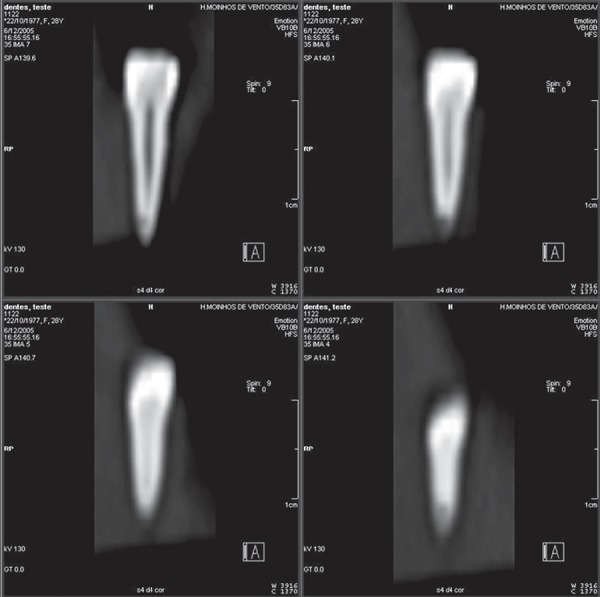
Series of coronal CT sections showing cavities on apical and middle thirds
